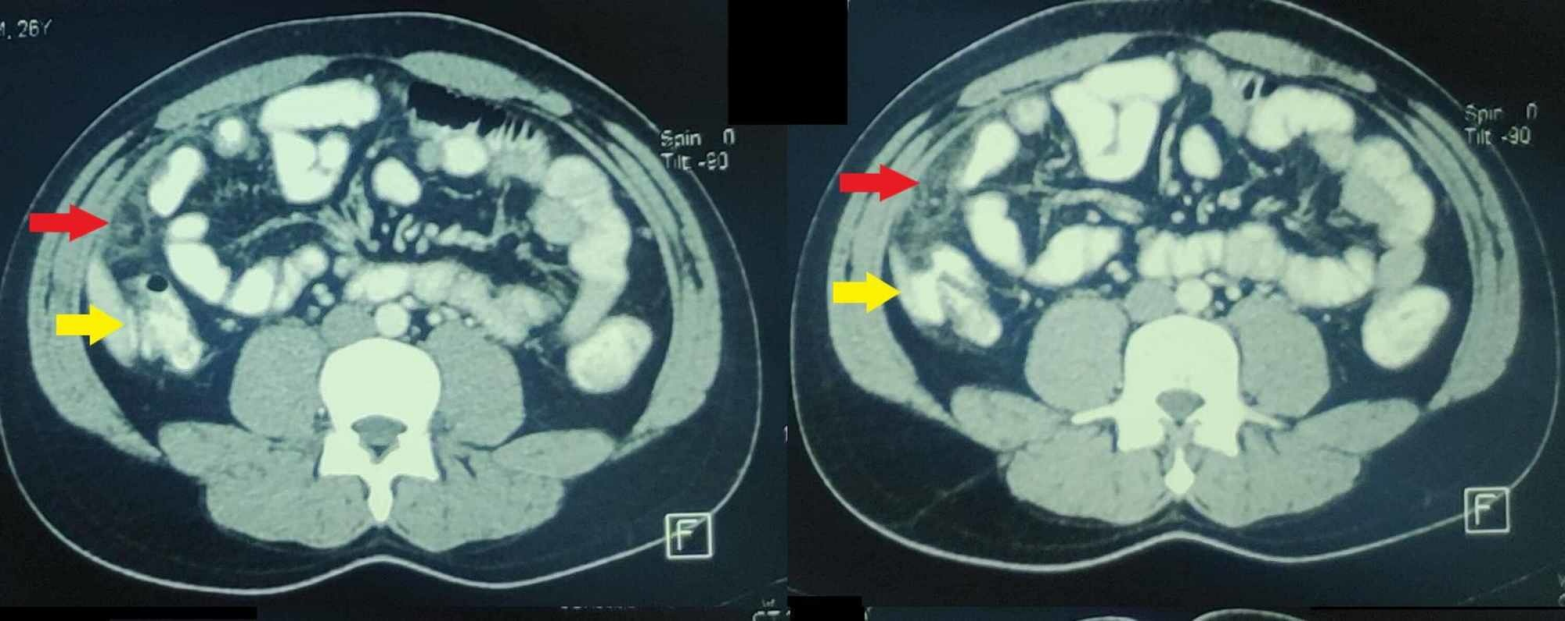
Axial sections of contrast-enhanced CT scan of the abdomen. The lesion produced by omental infarction (red arrows) can be seen anterior to the ascending colon (yellow arrows).
